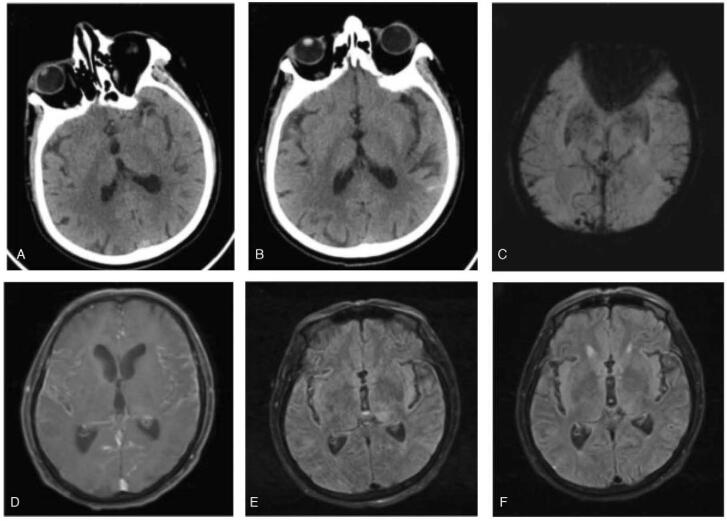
Imaging changes after the symptom onset (A) On the day of admission, CT indicates no bleeding. (B, C) On the 3th day following admission, CT and SWI indicates subarachnoid hemorrhage. (D, E) On the day of admission, MRI indicates extensive enhancement of the meanings, abnormal signals in the left thalamus and insula. (F) After 7 days of treatment, MRI indicates the thalamus and insula lesions were reduced. MRI = magnetic resonance imaging.
